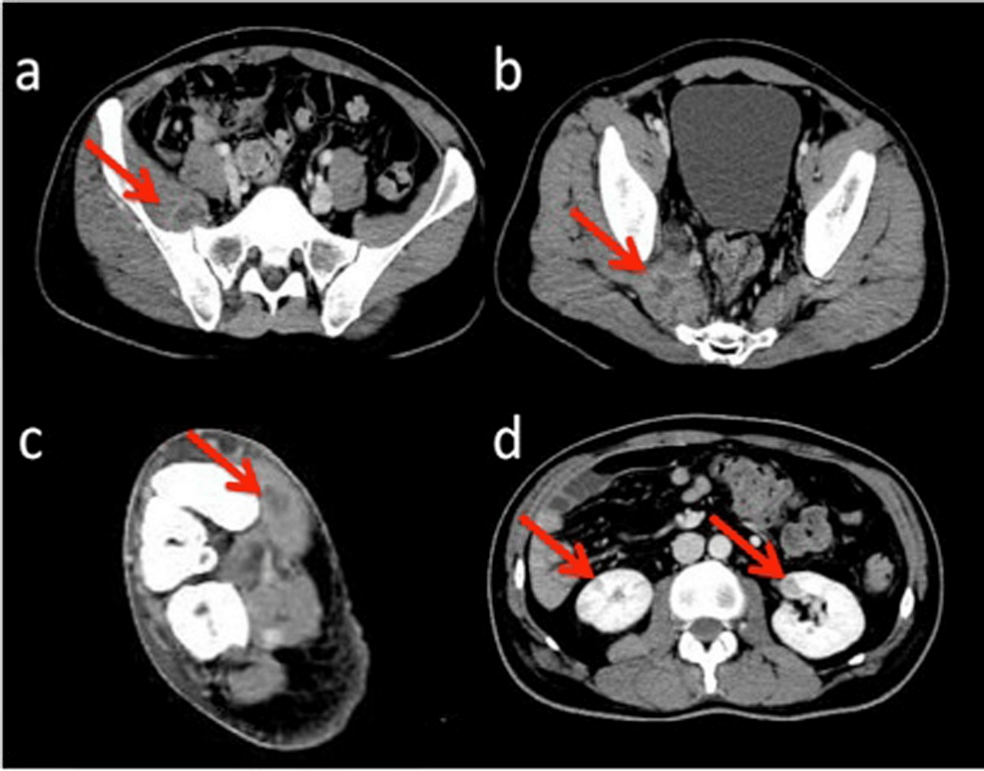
Contrast computed tomography scan at admission. (a: iliopsoas muscle, b: piriformis muscle, c: plantaris muscle, d: renal infarction) Arrows show abscesses.
